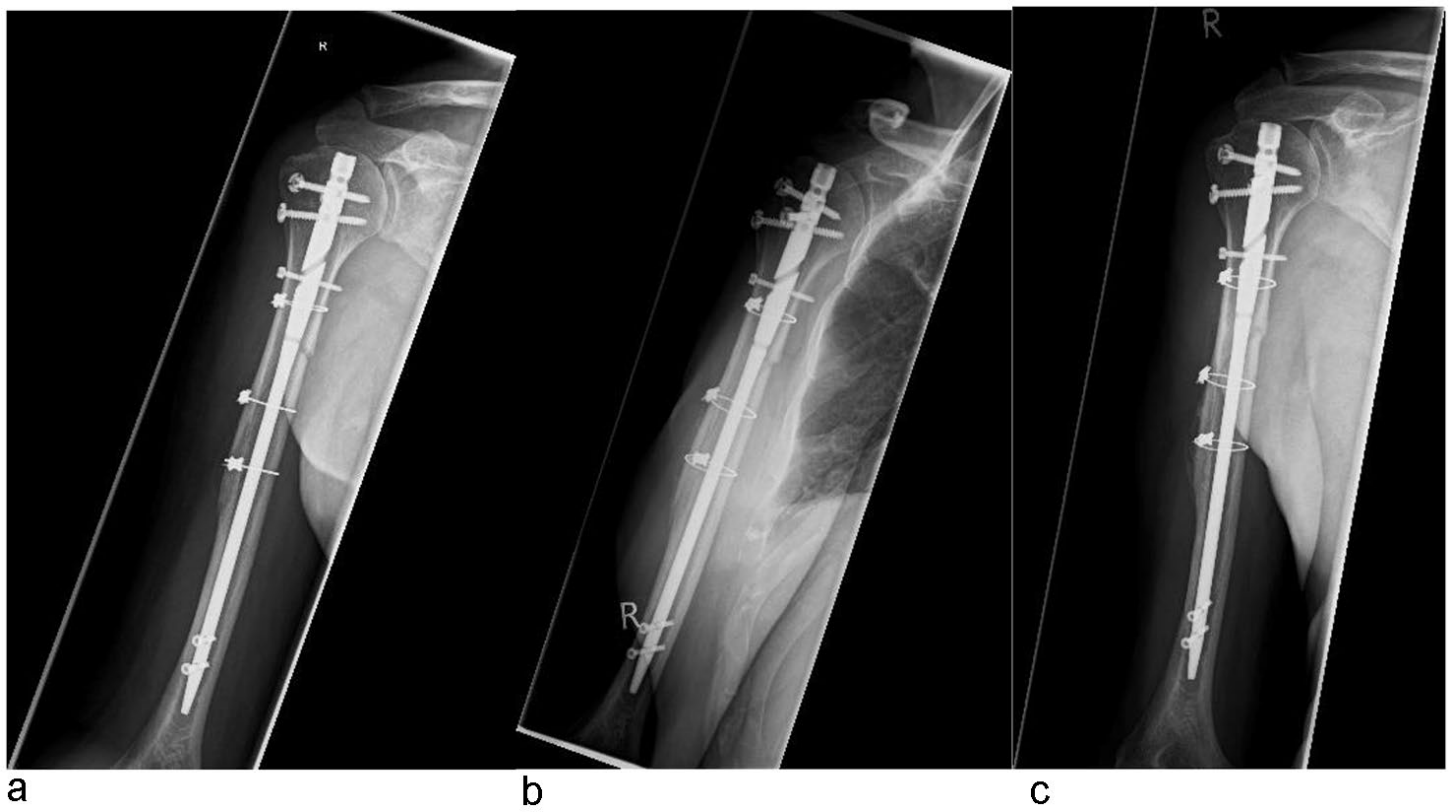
Healing process of the B2 fracture from Fig. 2. a The fracture gap is still clearly visible in the radiological X-ray check 6 weeks after surgical treatment. b Callus formation begins 12 weeks after operation. c Healing 6 months after the operation
